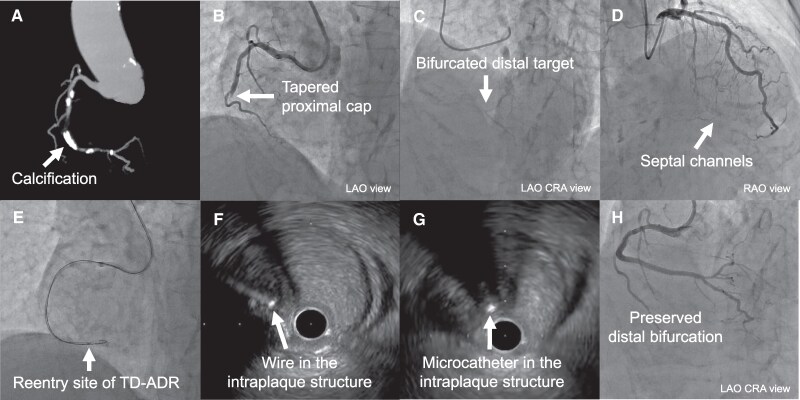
(*A*) CT angiogram highlighting lesion calcification. (*B*) Right coronary angiography demonstrating a tapered proximal cap. (*C*) Left coronary angiography showing a bifurcated distal target. (*D*) Left coronary angiography showing feasible retrograde options. (*E*) Re-entry site of tip detection-antegrade dissection and re-entry (TD-ADR). (*F*) A Conquest Pro 12ST guidewire in the intraplaque structure. (*G*) A microcatheter in the intraplaque structure. (*H*) Final angiogram showing a preserved distal bifurcation.
